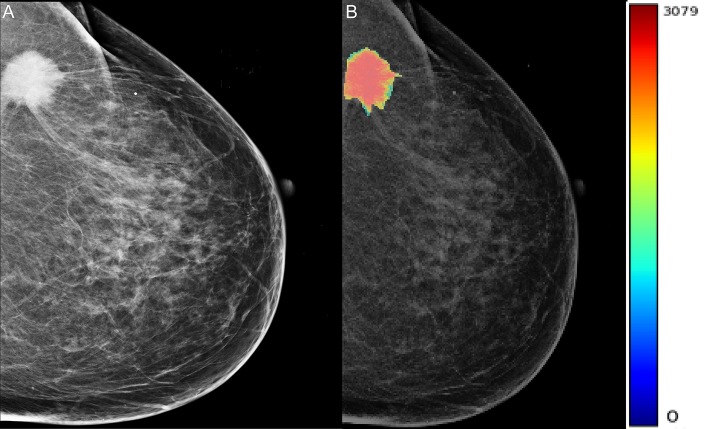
The woman, 60 years old, had triple-negative breast cancer, indicating low levels of neoplastic infiltrating lymphocytes in her left breast. (A) Oblique X-ray of the left mid lateral shows lobulated and partial burr mass of the left breast (arrow). (B) The X-ray image with a color overlay map of mass density indicates mass uniform and unsmooth.
